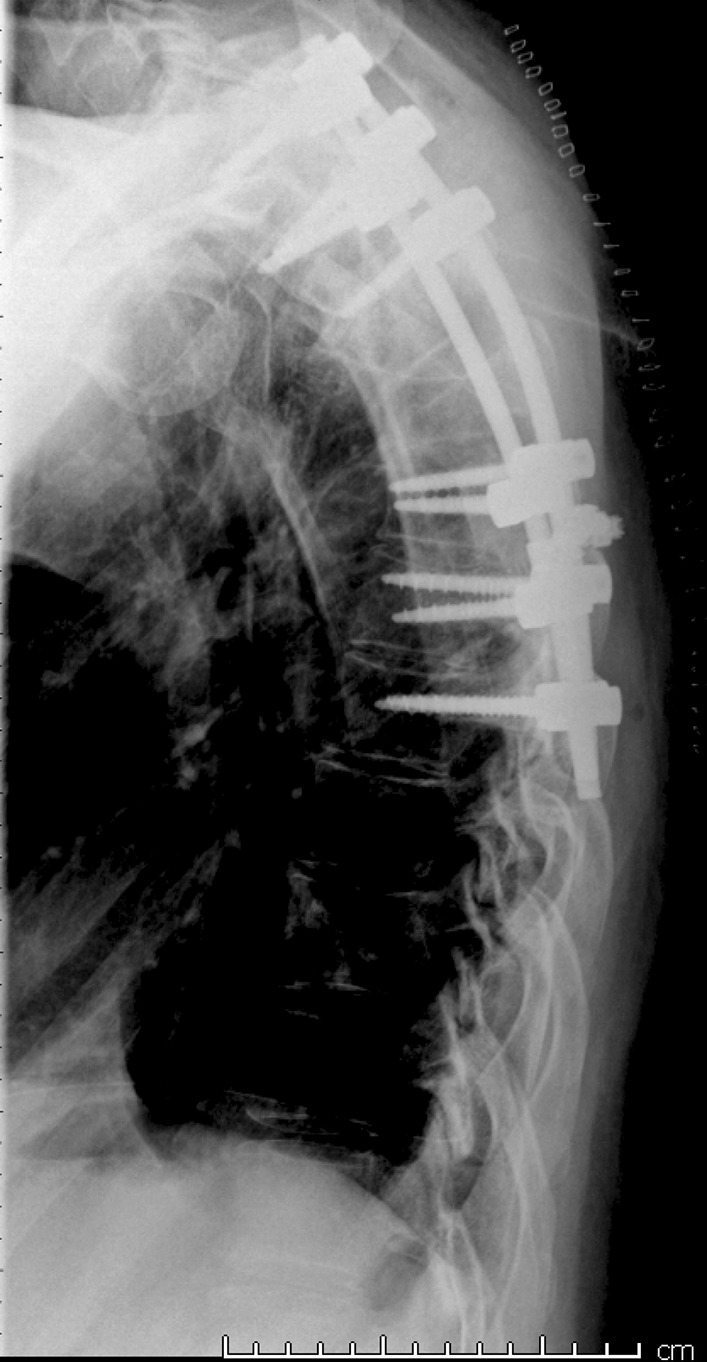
Chest x-ray of postoperative situation
